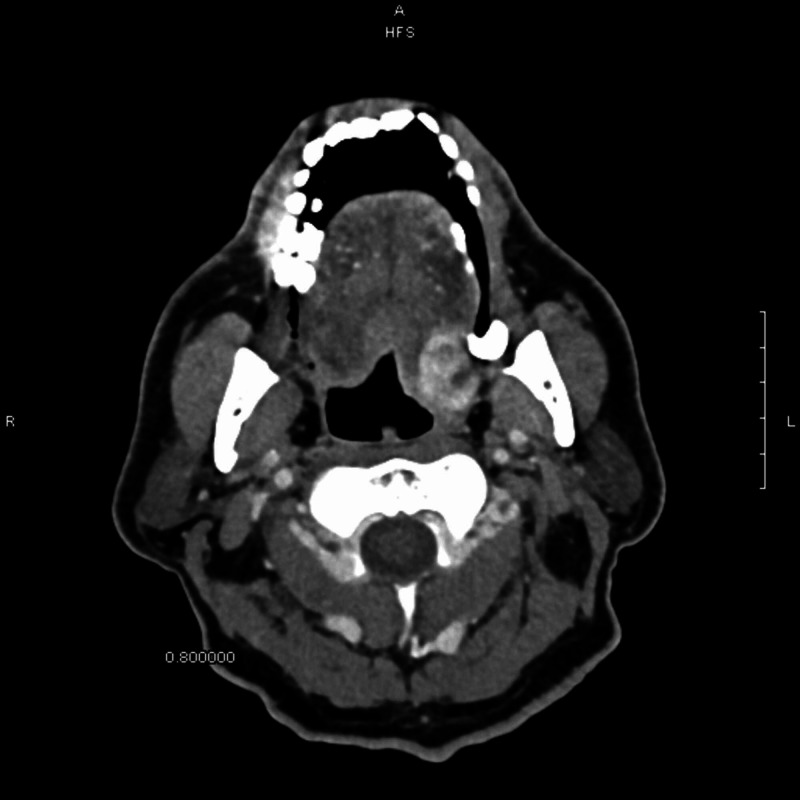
Surveillance head CT showing a suspicious lesion on the left base of tongue.
